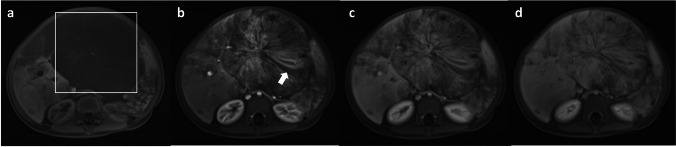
Magnetic resonance (MR) images of a 2-year-old girl with biopsy-proven hepatic angiosarcoma. a Unenhanced axial MR T1-weighted (T1W) scan showing a large left lobe mass with homogeneous intermediate T1W signal, limiting characterisation. b–d On the axial post-gadolinium contrast-enhanced arterial phase (b), portal venous phase (c), and delayed phase (d) MRI of the liver, the left lobe mass shows a progressive centrifugal and variegated enhancement pattern, with hypoenhancing striated areas (arrow in b), which possibly represent fibrous septations. Such enhancement pattern is highly suggestive of angiosarcoma. The white box in a demarcates the area imaged in the contrast-enhanced ultrasound in Fig. 3. Reprint with permission: [85]
